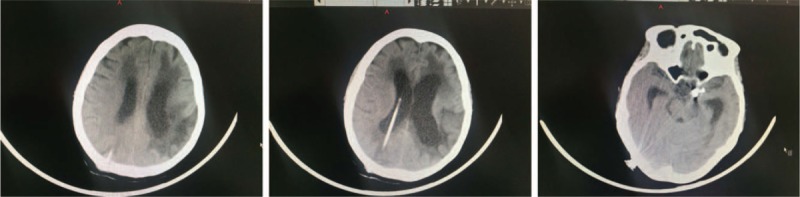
Head CT showed relief of hydrocephalus after ventriculoperitoneal surgery. CT = computed tomography.
